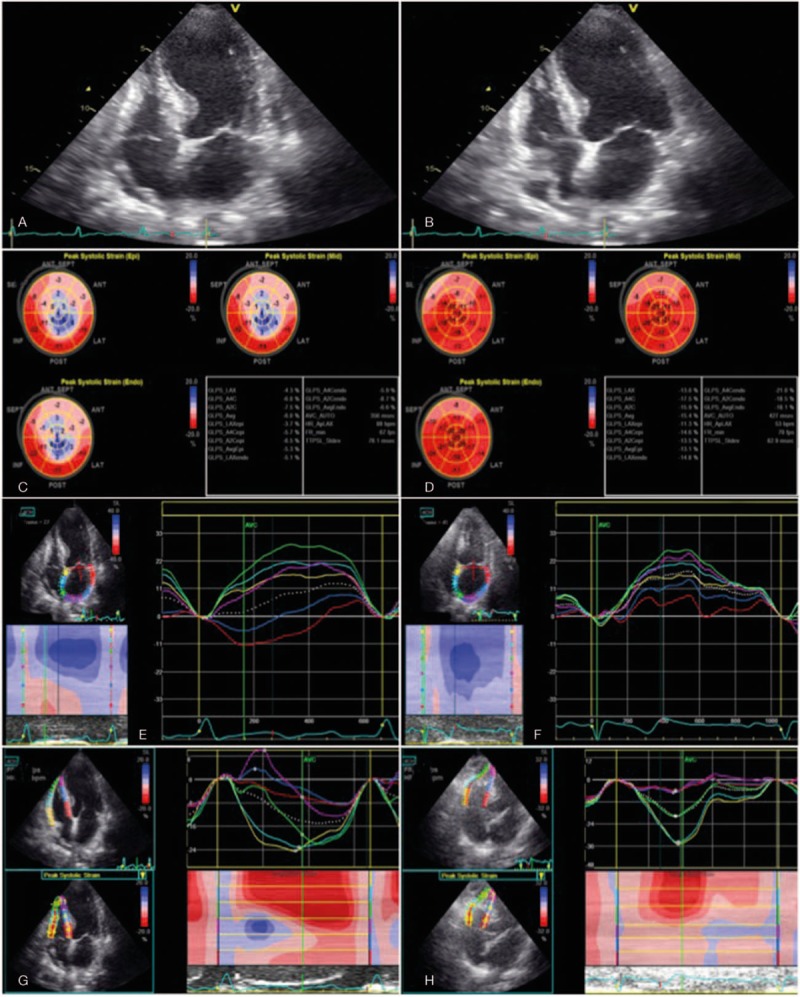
Echocardiograms: Two-dimensional echocardiograms show diffuse hypokinesis with normal contraction of the basal segments only at systole (A) compared with diastole (B). Myocardial deformation images: Left ventricular global longitudinal strain (C) and maximal left atrial strain (representative image from apical 4-chamber view, E) show reduced systolic strain on the 8th day, which improved on the 70th day (D and F, respectively). The right ventricular free wall strain values were within normal limits in both acute stage (G) and follow-up (H) studies. The averaged left atrial strain values are expressed as a white dashed line. The strain values of the 3 right ventricular free wall segments were averaged to obtain right ventricular free wall strain. LA = left atrium, LV = left ventricle, RA = right atrium, RV = right ventricle.
